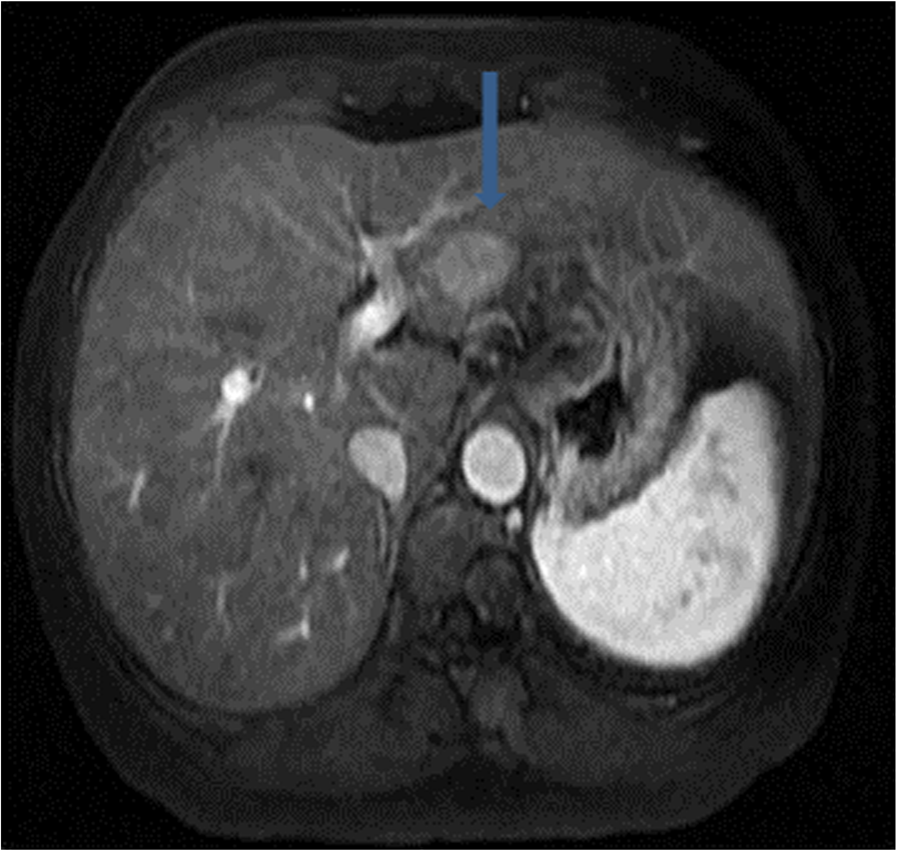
MRI of the liver in a patient with chronic liver disease. Arterial phase extracellular Gd-chelate-enhanced MRI demonstrating a T1 and T2 hyperintense sphenoid lesion of 1.8 cm (*arrow*) in diameter in the Sg 3 which is enhanced in the early phase
